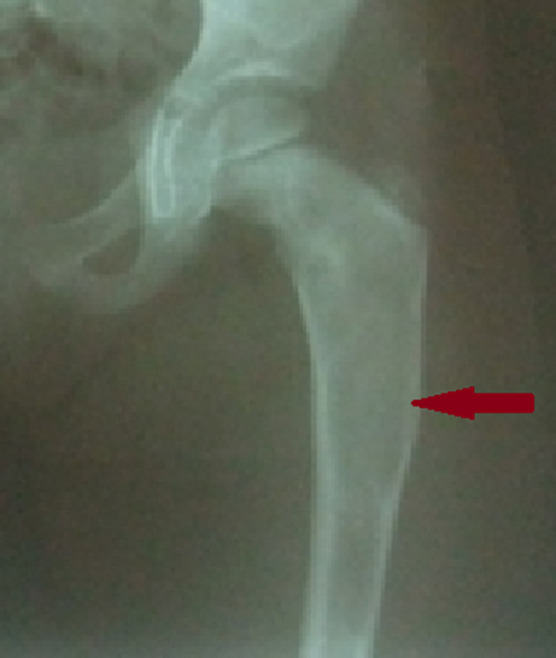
radiographie standard de la hanche de face révélant une lésion ostéolytique de l'extrémité supérieure du fémur gauche
